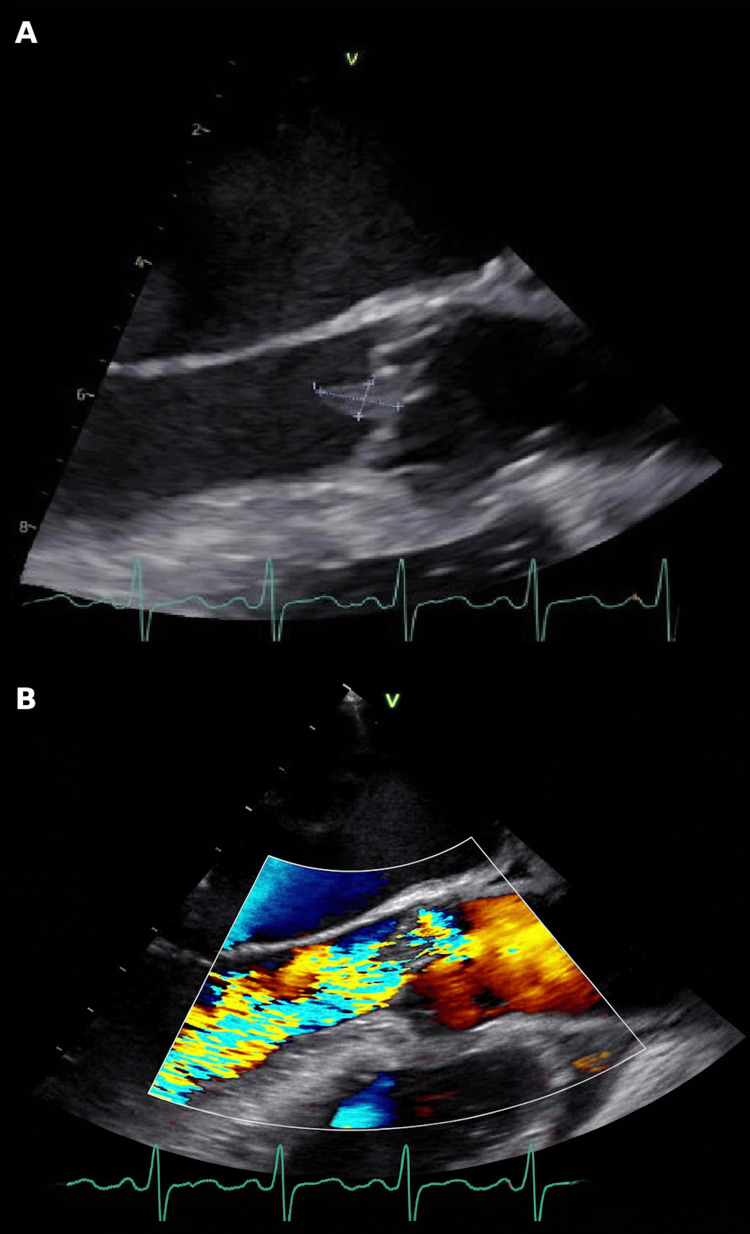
Transesophageal echocardiogram. (A) Mid-esophageal long-axis view demonstrating a 1.0 × 0.5 cm mobile vegetation on the ventricular surface of the left coronary cusp of the aortic valve.
